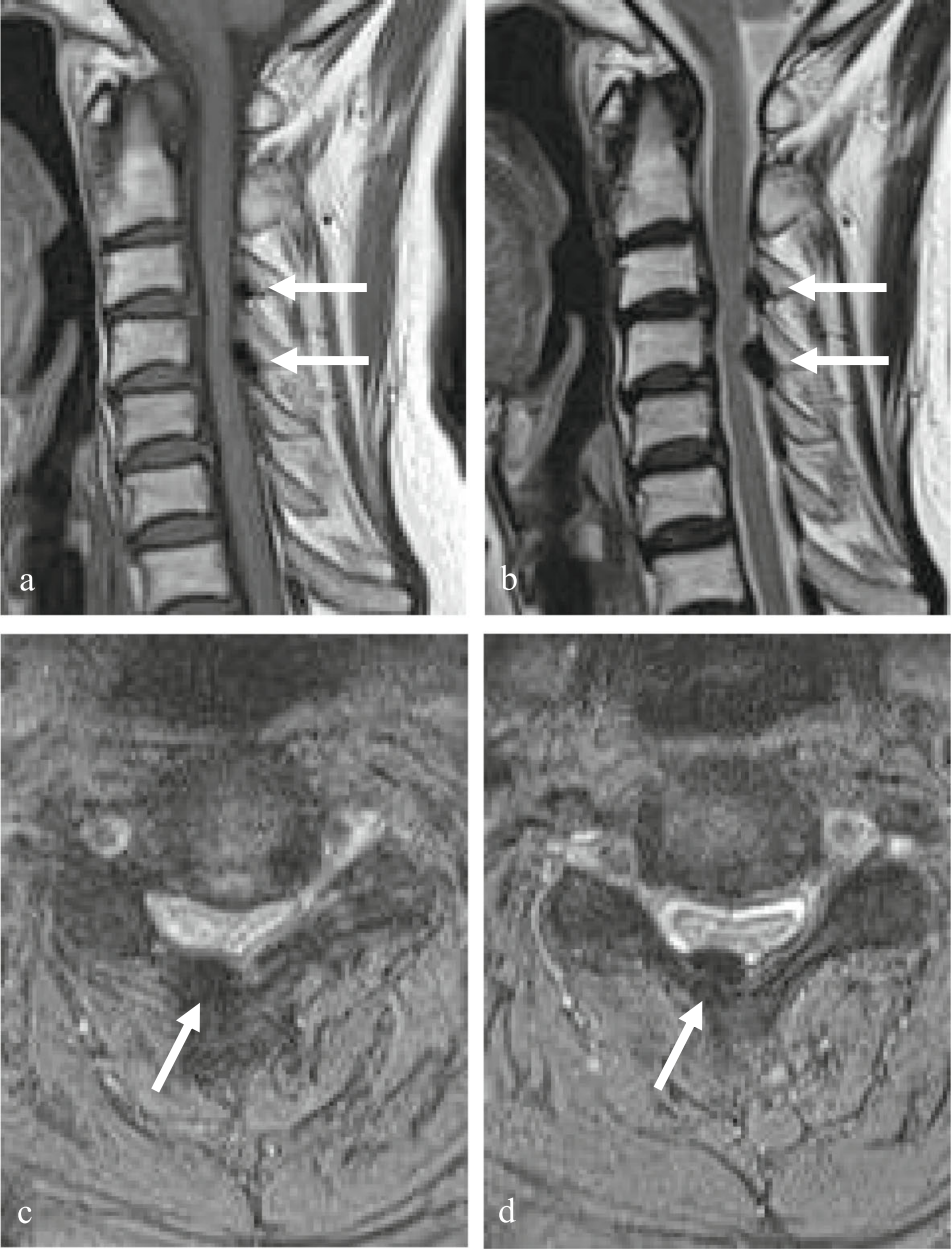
Sagittal (a and b) and axial (c and d) MRI showed spinal cord compression by dark round lesions at the laminae of C3 and C4 (white arrows)
